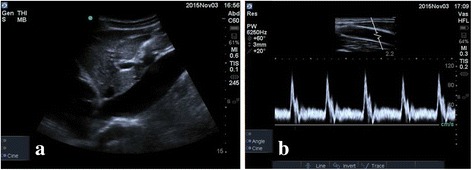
Example IVC and carotid artery ultrasound images. a IVC diameter measurements were taken 3-5 cm distal to the IVC-right atrial junction. b Common carotid artery diameter and Doppler flow were measured 2 cm proximal to the carotid bulb
